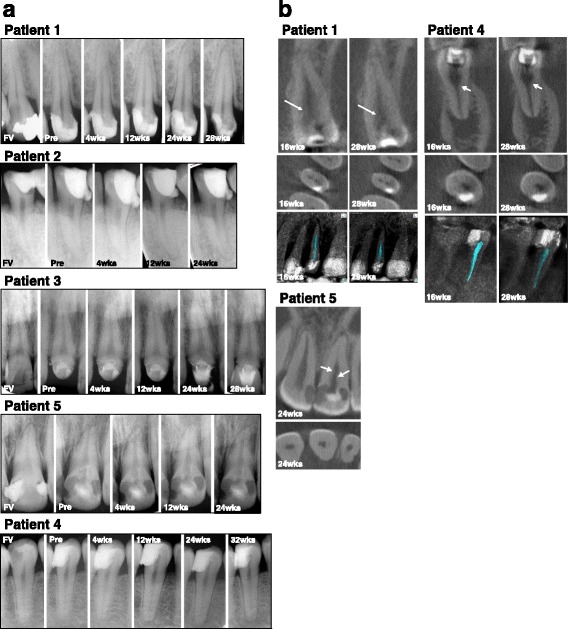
Radiological analyses. a X-ray photographic analysis to show the changes and evolution of periapical tissues and apical and/or lateral dentin formation in the root canal at the first visit (*FV*), pre-transplantation just before cell transplantation (*Pre*), and 4, 12, and 24/28 weeks (*wks*) after autologous transplantation of MDPSCs with G-CSF in pulpectomized teeth in five patients. No significant changes were seen in the periapical areas, except in patient 4 who showed widening of periodontal ligament space at 12 weeks and periapical radiolucency at 24 and 32 weeks. Patient 2 preoperatively had periapical radiolucent lesion decreased in area size with a little radiographic periodontal ligament space widening at 24 weeks. b Cone beam computed tomography evaluation of apical/lateral dentin formation in the root canal in coronal and axial slices in three patients at 16 and 28/32 weeks. *Arrows* indicate newly formed dentin. The 3D cone beam computed tomography images by the OsiriX program demonstrated a decrease in low-density areas at 28 weeks compared with 16 weeks
